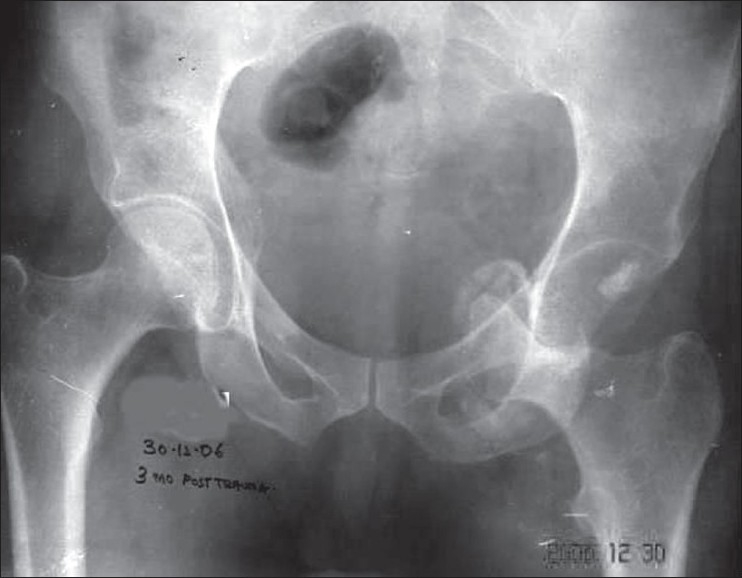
Preoperative anteroposterior X-ray of pelvis with both hips showing intra pelvic dislocation
